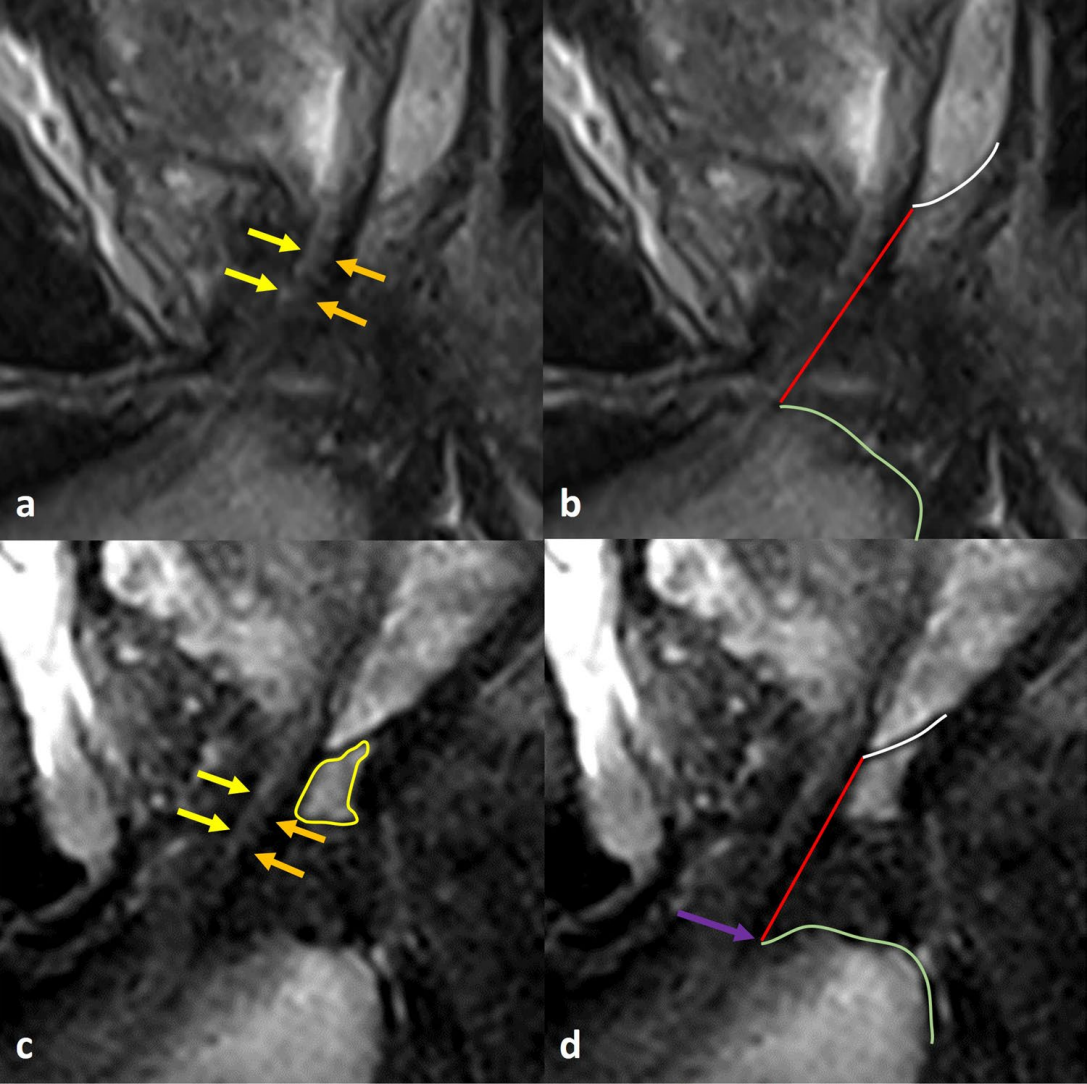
Critical steps in membranous urethral length (MUL) measurement on midsagittal MR images. a, b A prostate cancer patient with sagittal T2w images on preoperative MRI. In MUL measurement, critical steps need to be distinguished: (1) the hyperintense lumen (yellow arrows) and the hypointense dorsal part (orange arrows) of the membranous urethra need to be identified. (2) The line of the membranous urethra measurement should be placed dorsally and parallel (red line, MUL). (3) The upper border of the measured membranous urethra intersects with the prostate apex, defined as the lower border of the peripheral zone at the dorsal side (white line). (4) The lower border the measured membranous urethra intersects with the penile bulb, the bulb of the corpus spongiosum (green line). c, d Another prostate cancer patient with sagittal T2w images on preoperative MRI. Notice the difference between hyperintense signal of the peripheral zone and retroprostatic part of the rectovesical space (yellow delineation). Also notice base of the penile bulb can be slightly curved (purple arrow), extending the MUL slightly. The measurement (red line) was 20 mm in this case
